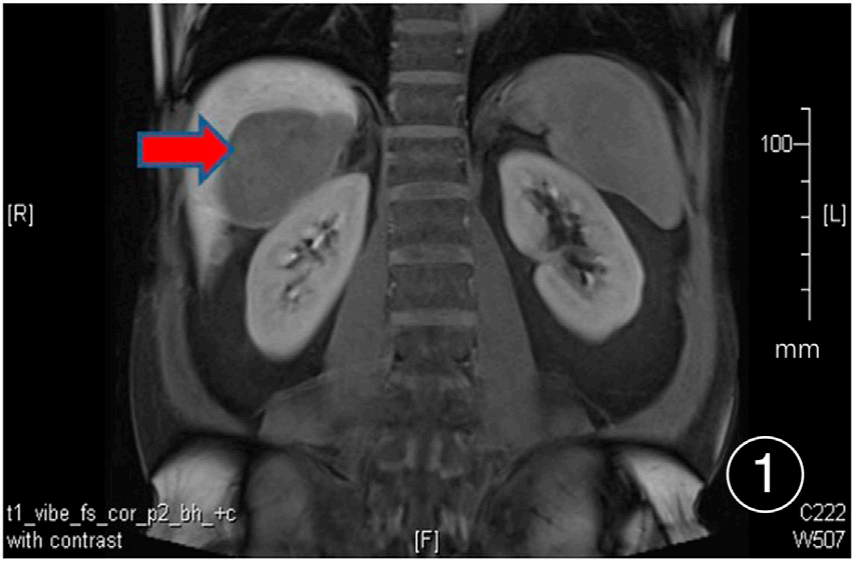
PET/CT shows multiple nodular and lump-like abnormal signal lesions under the liver surface, the larger one is located in the space between the liver and the right kidney, about 85 mm × 57 mm in size.
